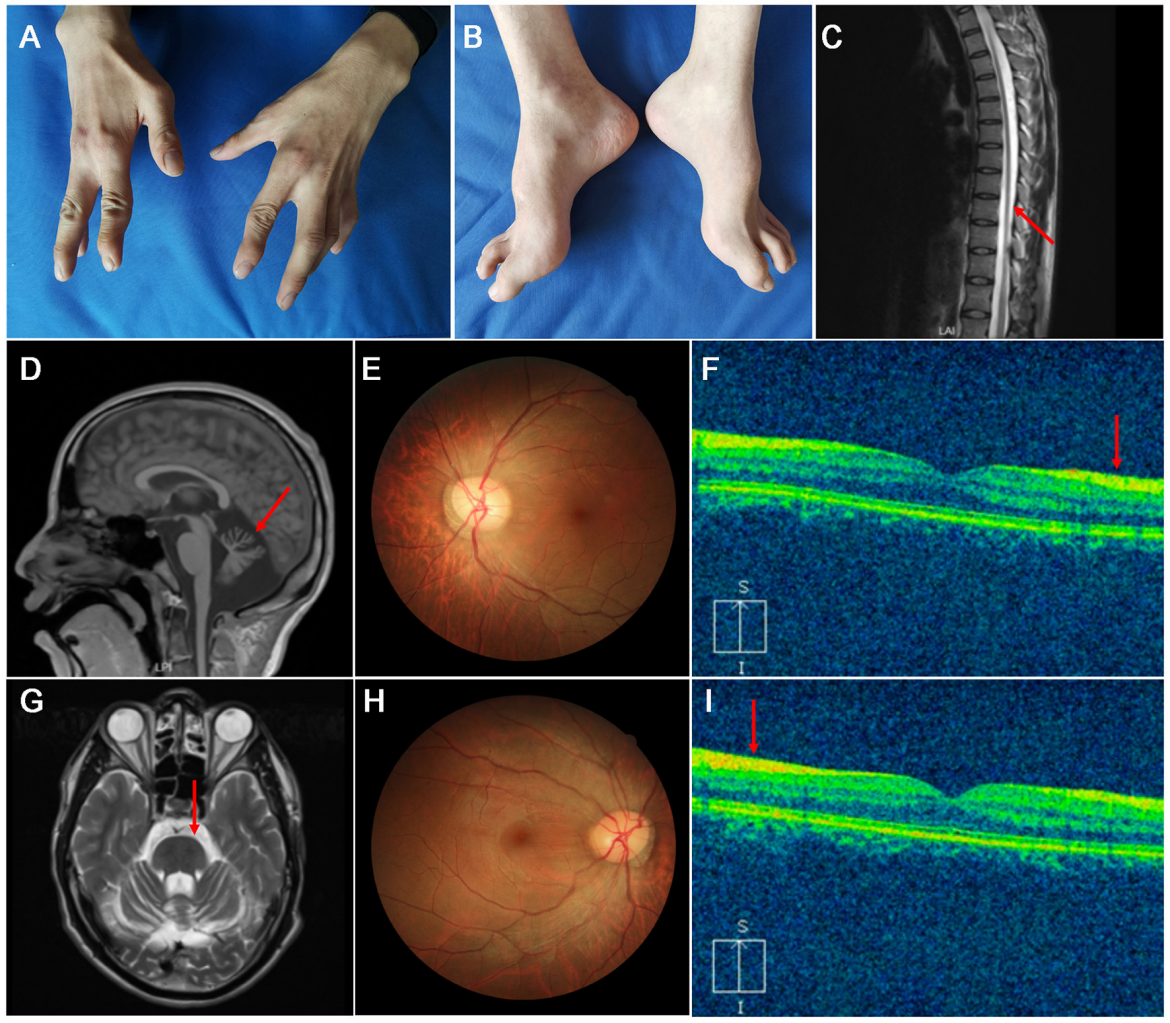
The clinical and neuroimaging features of the patient. (A,B) Neurological examinations show Hammer toes and pes cavus. (C) Thinning spinal cord in sagittal T2-weighted image (arrow). (D,G) The brain MRI shows the cerebellum atrophy in the sagittal T1-weighted image (arrow), hypointensities in the pons in the axial T2-weighted image (arrow). (E,H) Fundus photographs of the right and left eyes show swollen papilla with a combed aspect of the interpapillomacular region. (F,I) Optical coherence tomography imaging of the right and left eyes shows thickened RNFL (arrow).
